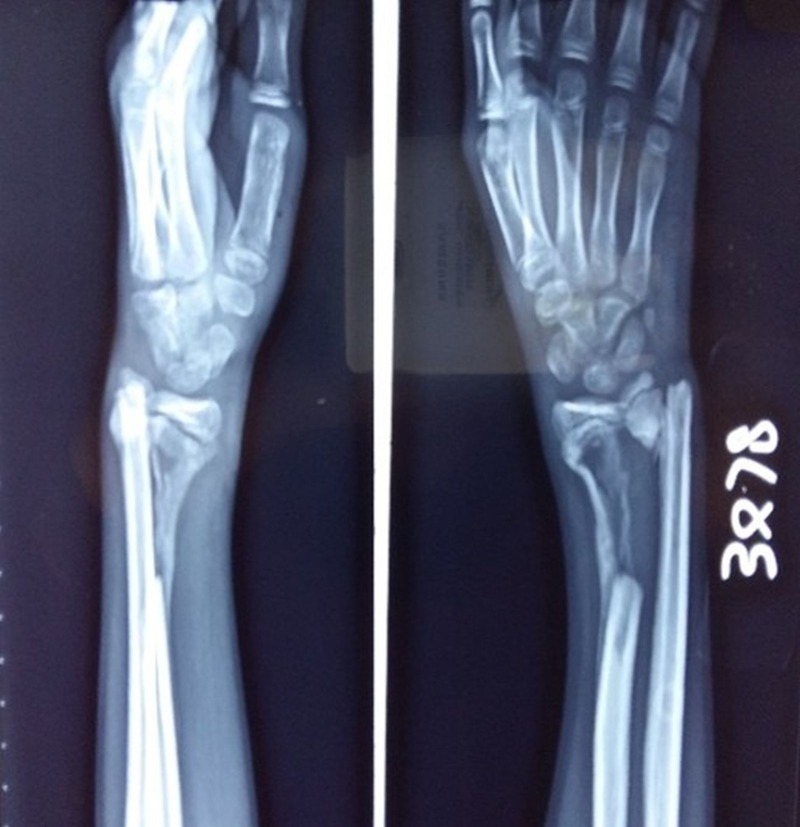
Anteroposterior and lateral radiographs showing the bone formation at four weeks.
